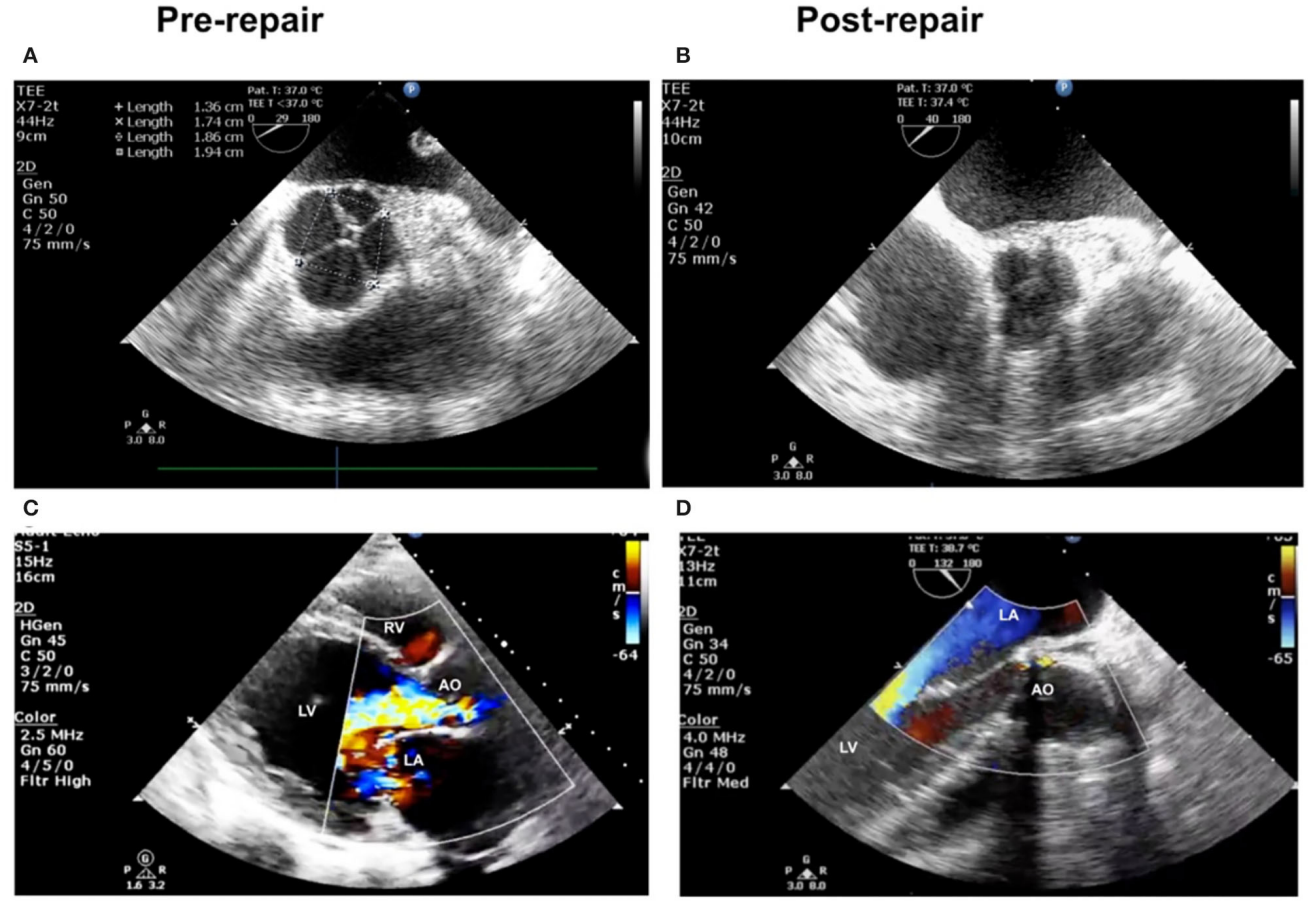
(A) Pre-operation: quadricuspid aortic valve (QAV). (B) Post-operation: QAV to tricuspidization aortic valve (TAV). (C) Transthoracic echocardiogram (TTE) demonstrated severe regurgitation before operation. (D) Post-operation image of transesophageal echocardiography (TEE) showed no regurgitation.
